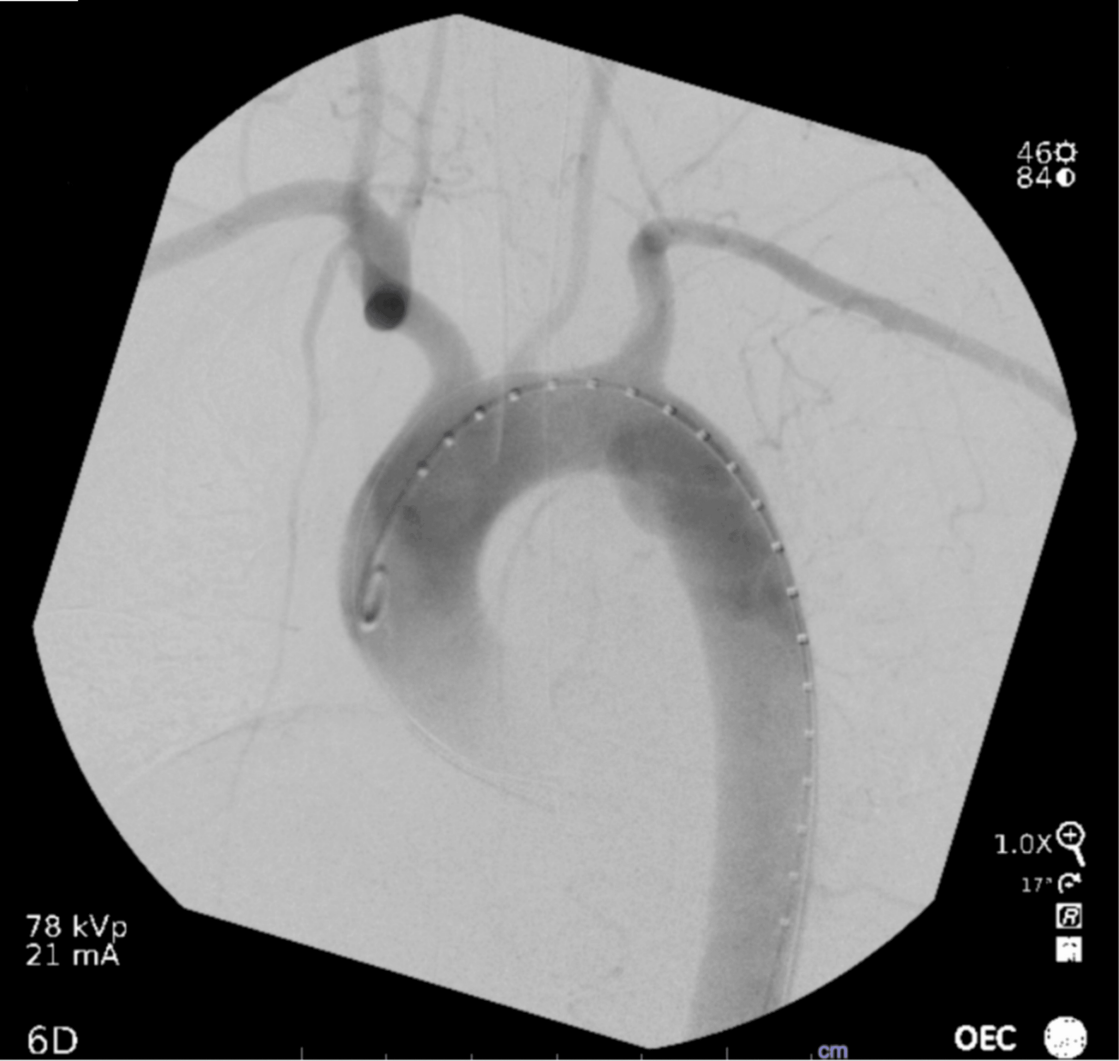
CT angiography with contrast visualizing the aortic arch and descending aorta preplacement of the stent.
